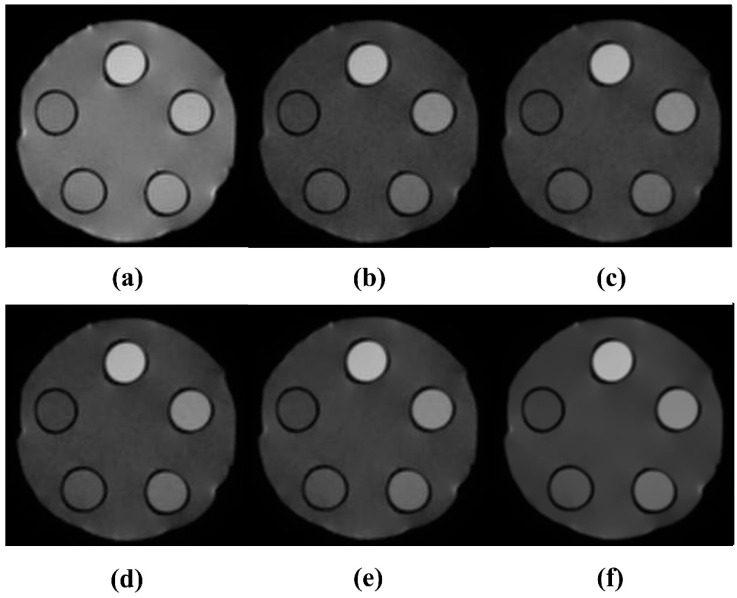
Comparison of diffusion-weighted magnetic resonance images using no algorithm, conventional algorithms, and the optimized non-local means algorithm: (a) b = 600 s/mm^2^, no algorithm; (b) b = 1000 s/mm^2^, no algorithm; (c) median; (d) Wiener; (e) total variation; (f) optimized non-local means.
